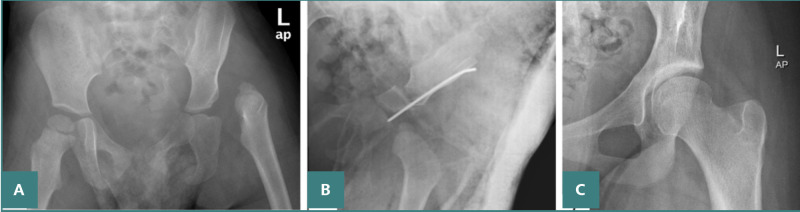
Post-operative X-ray of a patient following open reduction. A: Salter osteotomy, B-C: Anteroposterior left hip X-ray at the age of 11.
